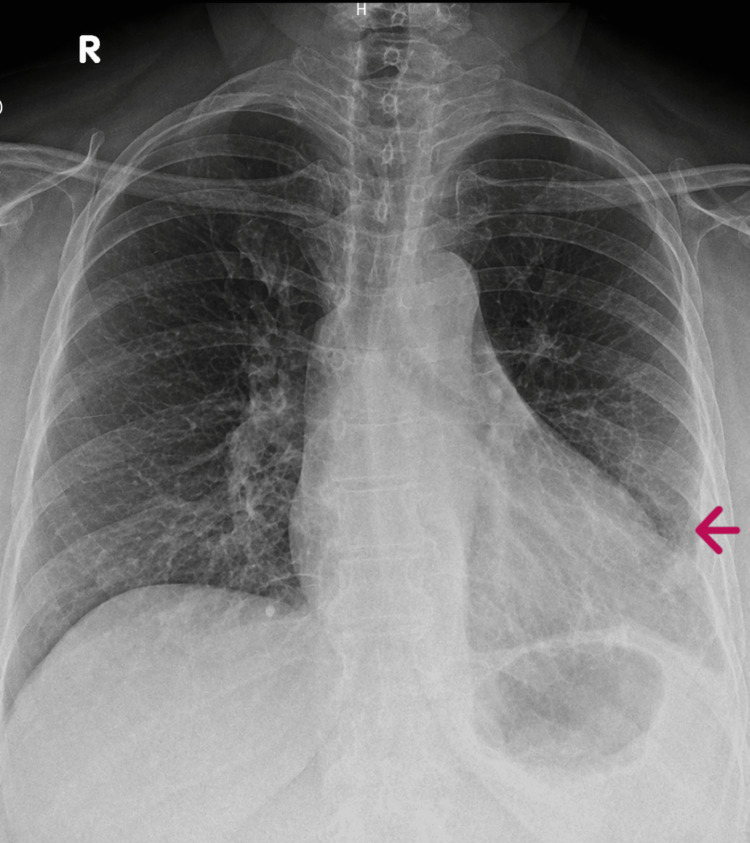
Follow-up chest radiograph performed four months later demonstrating significant resolution of the left-sided pleural effusion following conservative management.
